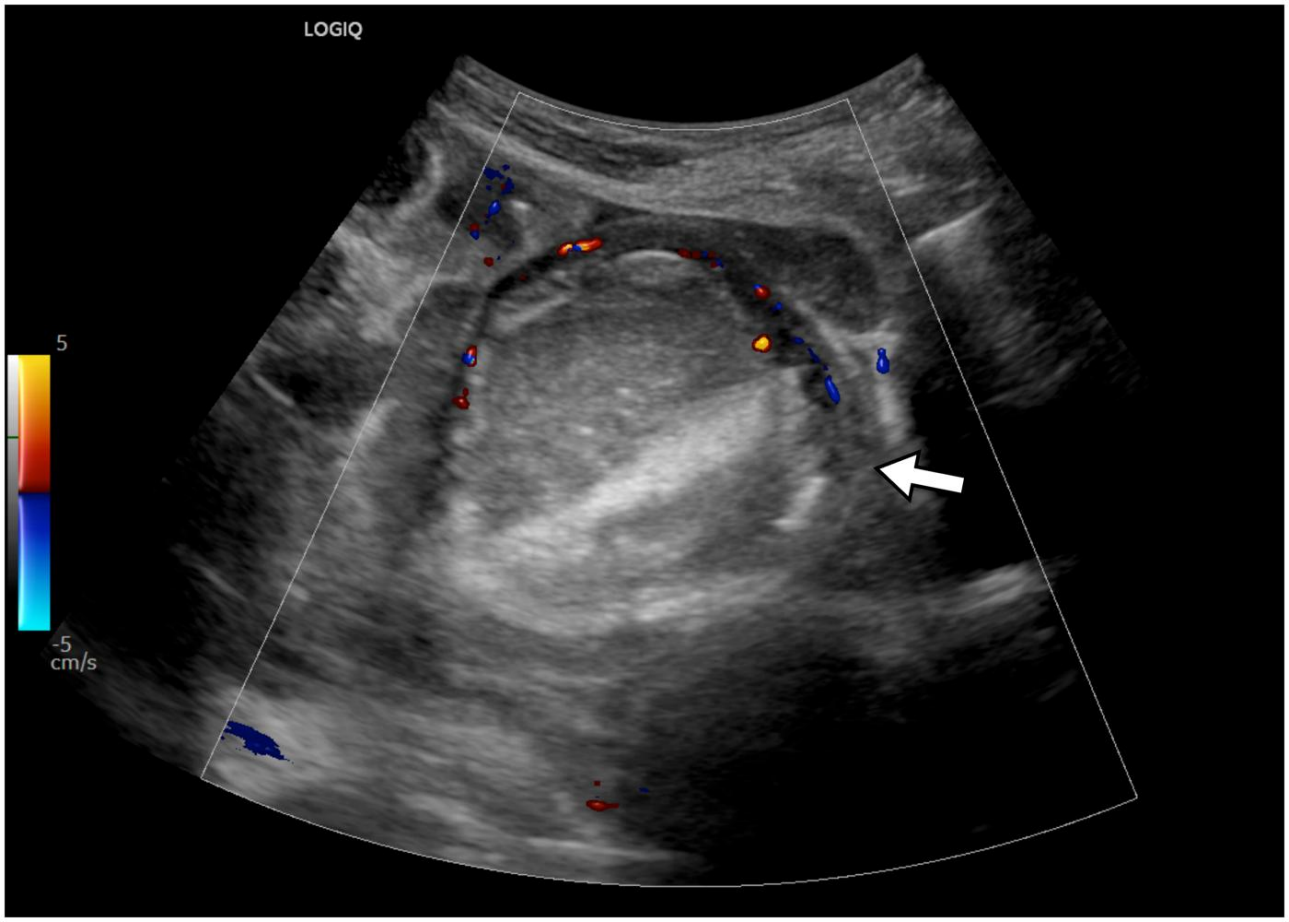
Ultrasonogram of the pelvis shows a well-defined, hyperechoic mass in the left adnexa, without internal vascularity. The left ovarian stroma is demonstrated at the periphery, showing vascularity on colour Doppler (arrow).
